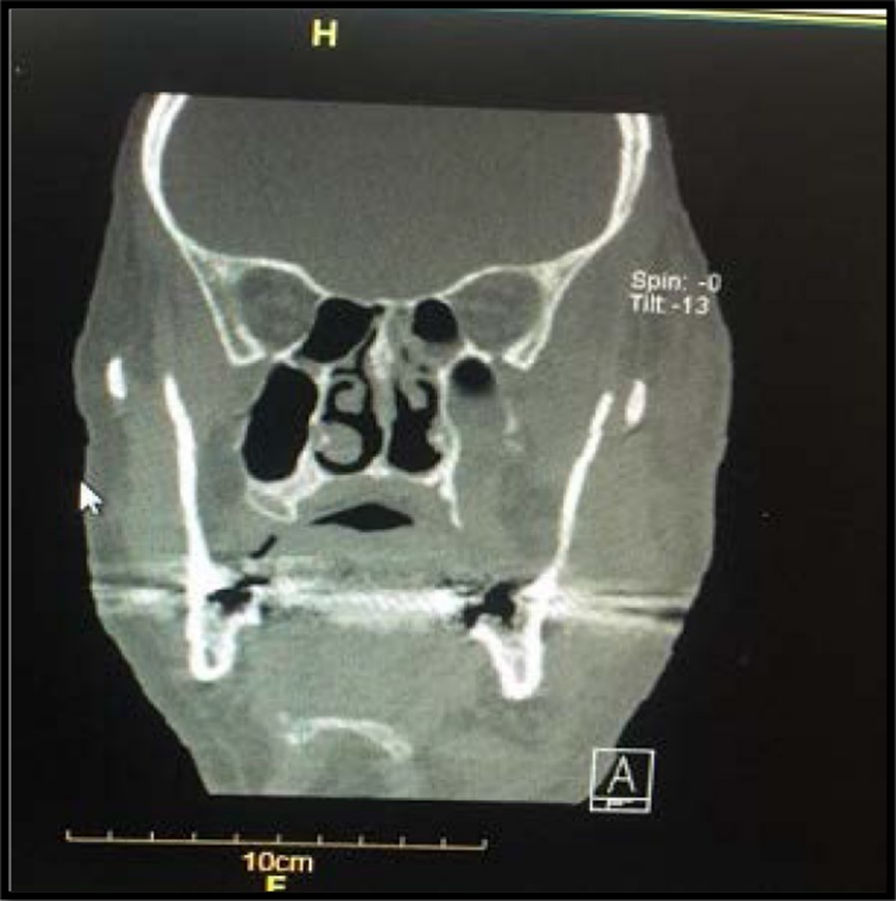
CT scan shows involvement of the left ethmoid, sphenoid, maxillary and paranasal sinuses in a patient with mucormycosis
